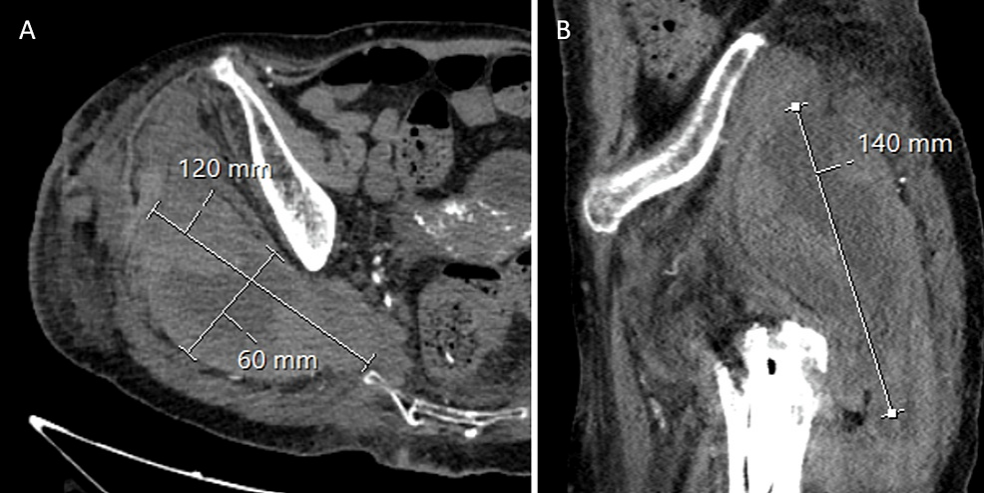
Axial (A) and sagittal (B) CT scans showing a large hematoma in the right gluteal region
